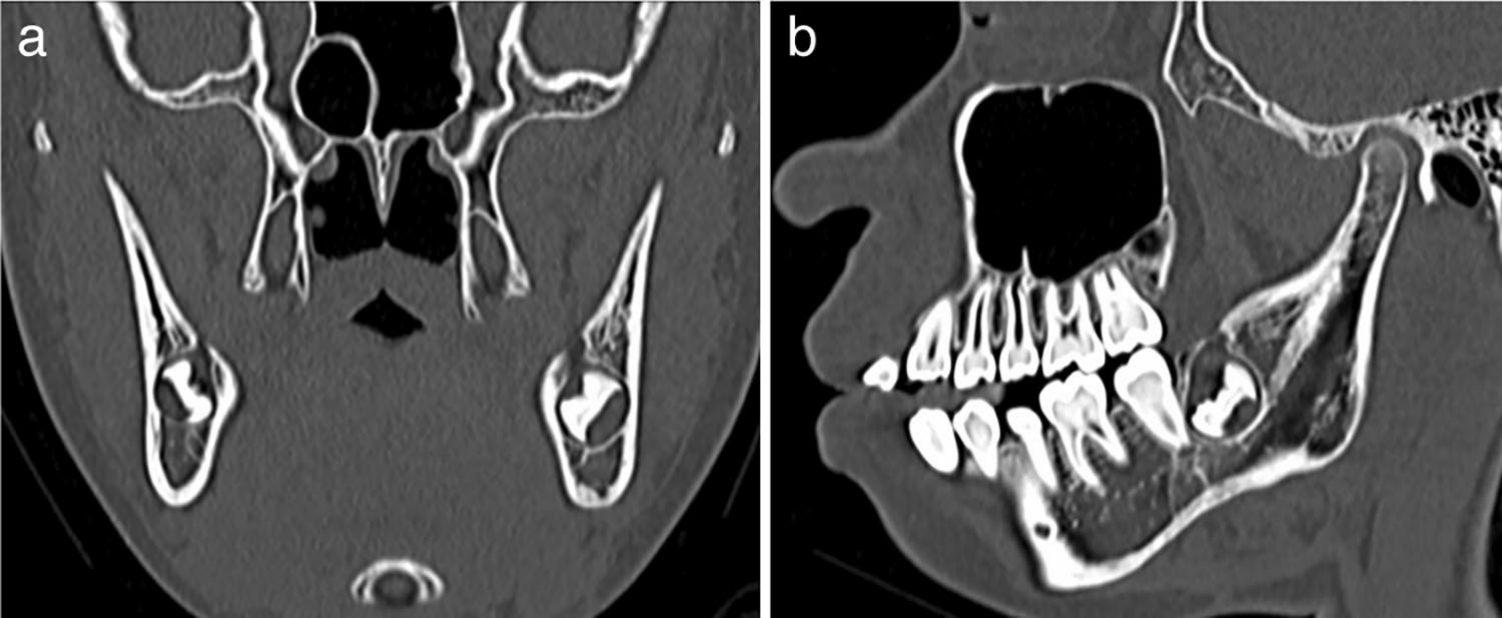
Follow-up computed tomography (CT) of the mandible at 1.5 years postoperatively showing no evidence of residual tumor or lesion recurrence with bone window settings (window width: 3000 HU; window level: 600 HU). The bone at the operated site shows complete healing. a Coronal CT image. b Sagittal CT image
